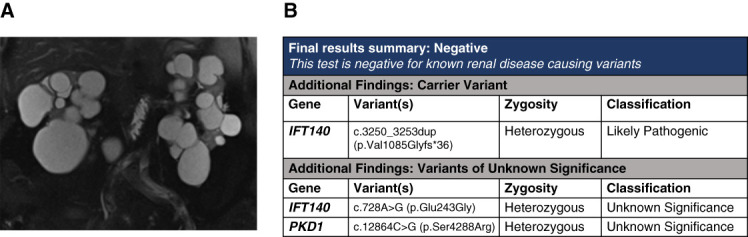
Imaging and genetic sequencing results of case 2. (A) Coronal T2-weighted abdominal MRI showing small kidneys with multiple cysts. (B) The patient has a carrier variant in *IFT140* and a VUS in *IFT140* and *PKD1*.
